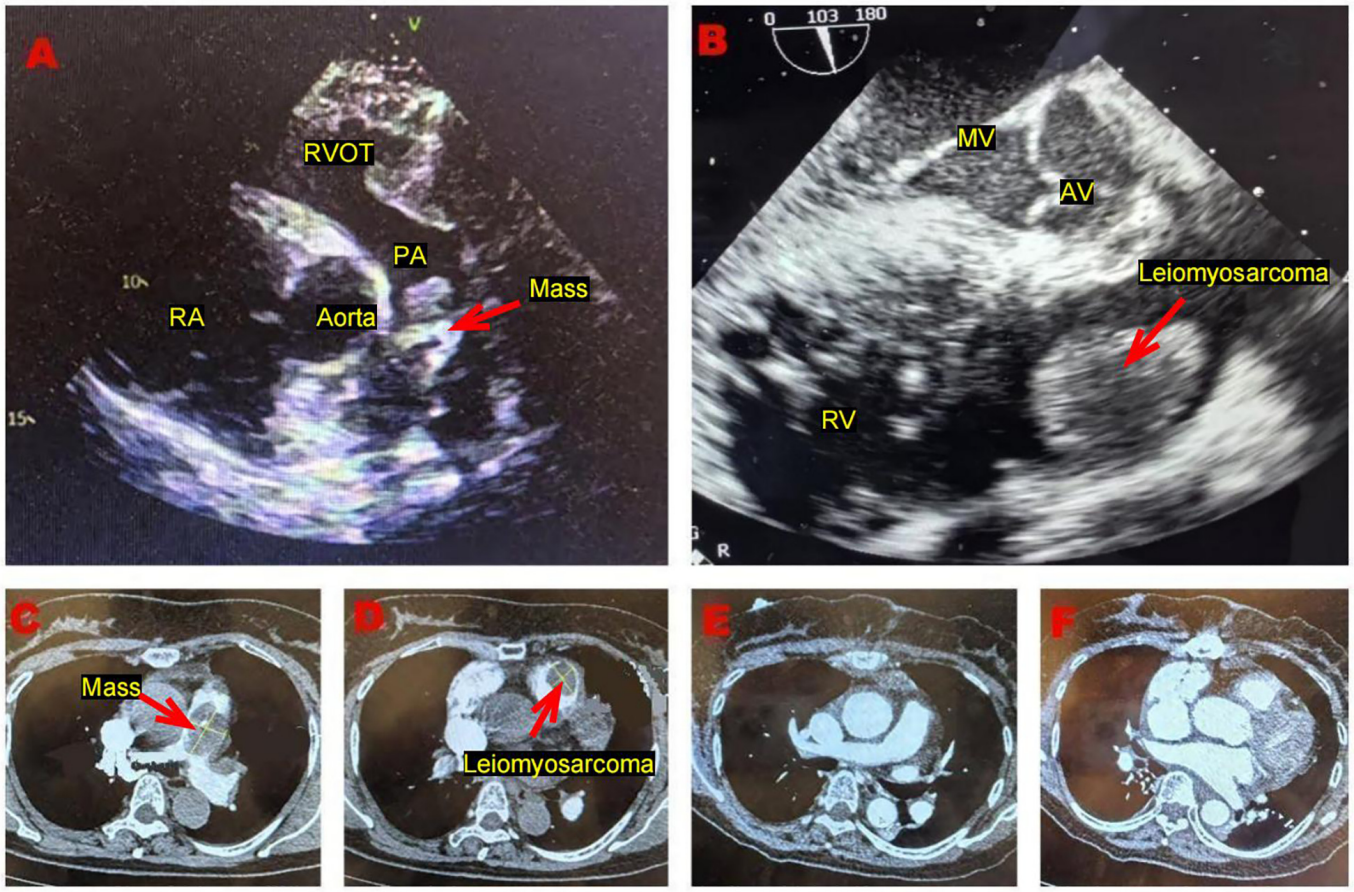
(A) Transthoracic echocardiography, performed in the short-axis view of the aorta, demonstrates an irregular isoechoic mass, situated within the main pulmonary artery. (B) Transesophageal ultrasound reveals an isoechoic mass with a regular shape situated in the atypical region of the right ventricular outflow tract. This mass appears to be connected to the anterior wall of the right ventricle via a pedicle. (C) Pulmonary artery computed tomography angiography reveals a patchy filling defect in the main pulmonary artery. (D) Pulmonary artery computed tomography angiography reveals a filling defect in the right ventricle outflow tract. (E,F) The postoperative pulmonary artery computed tomography angiography indicates no signs of residual tumor.
